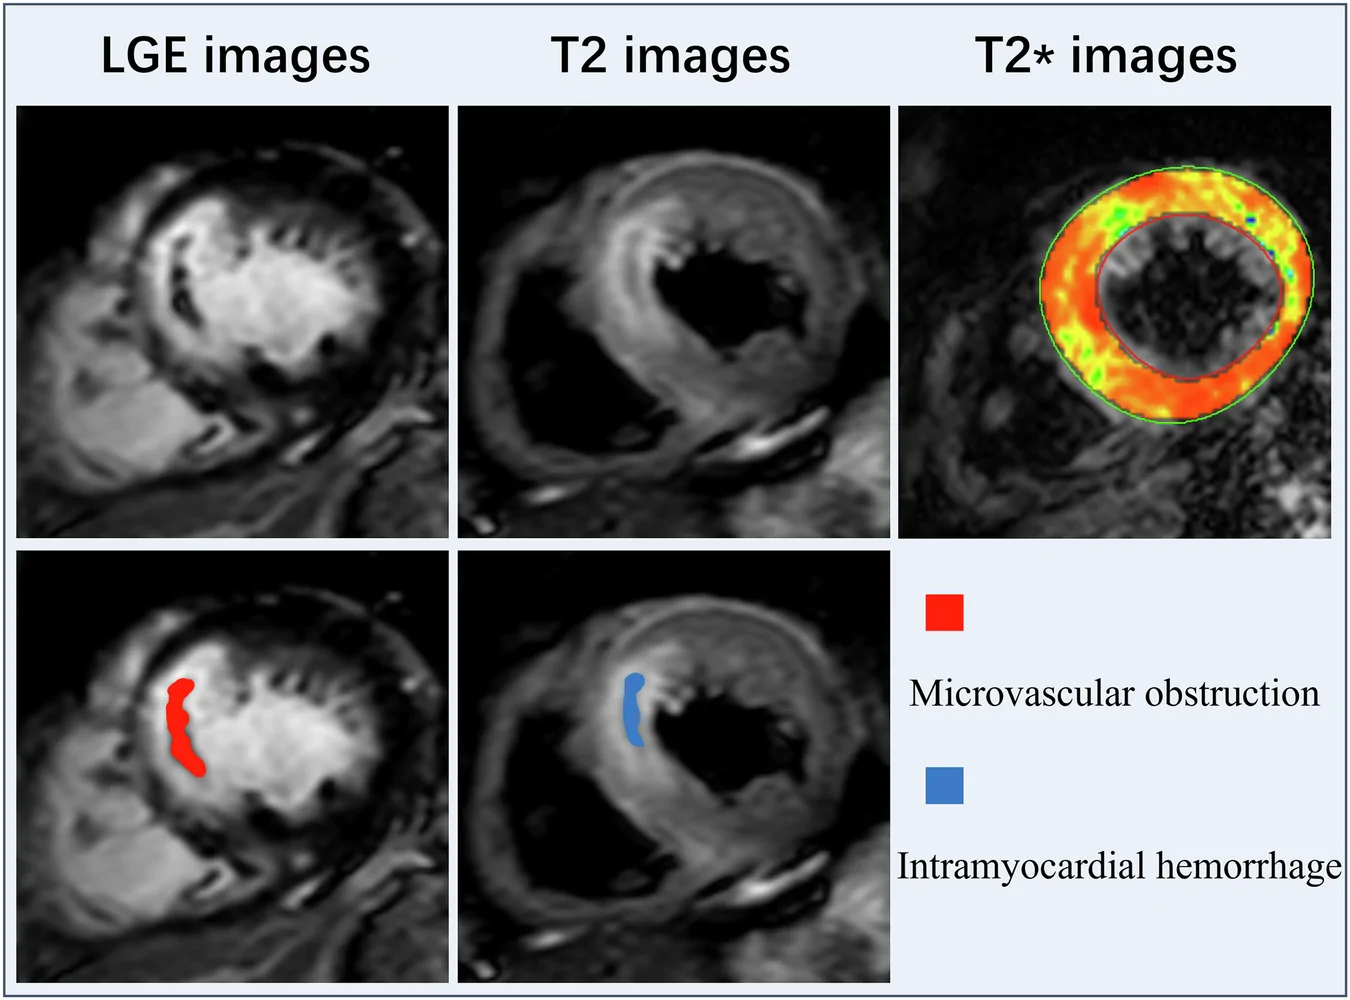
Example of microvascular obstruction and intramyocardial hemorrhage size assessed by cardiac magnetic resonance imaging. LGE, late gadolinium enhancement
